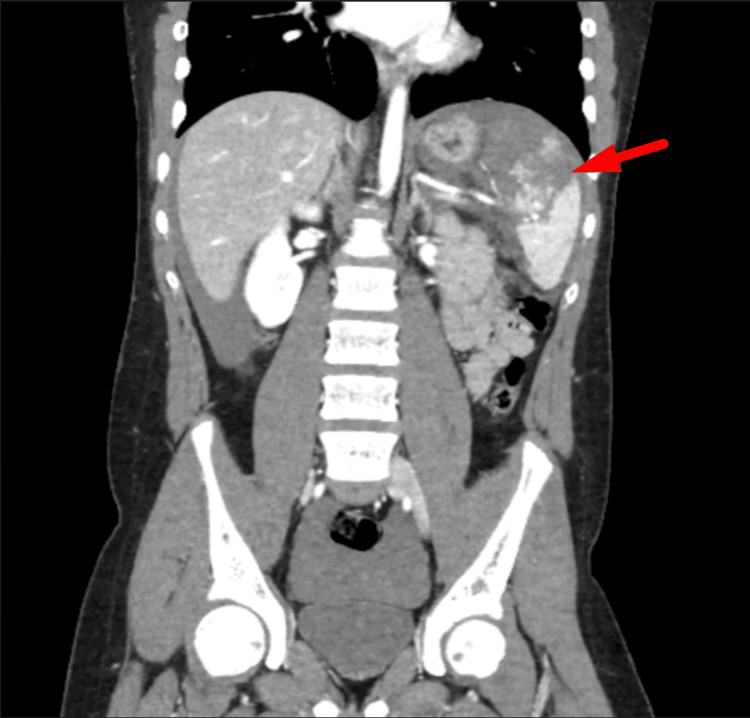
CT axial view demonstrating a grade 5 splenic laceration CT: computed tomography
